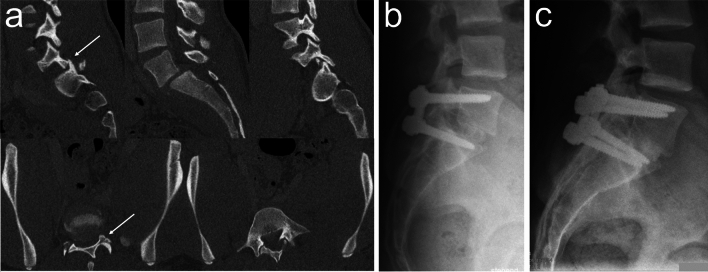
Lumbosacral dislocation injury (LSDI; Type 1 TLSI). A 16-year-old polytrauma patient sustained an LSDI following a skiing collision with a tree and presented with left-sided L5 radicular pain, sensory deficit, and mild L5 motor weakness (Grade 4/5). a Preoperative CT images demonstrating a fracture-dislocation of the left lumbosacral facet joint (*white arrows*), anterolisthesis of L5 on S1, and a fracture of the right S1 facet. b Postoperative lateral radiograph after L5-S1 instrumentation and circumferential fusion using autologous monocortical bone grafts and cancellous bone harvested from the posterior iliac crest. c Lateral radiograph at one-year follow-up. The patient reported no complaints and had already returned to skiing
